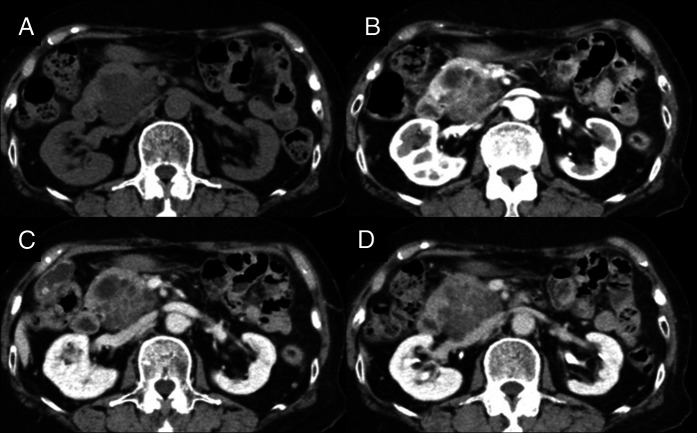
Abdominal dynamic computed tomography. (A) Plain scan, (B) late arterial phase, (C) portal venous phase, and (D) equilibrium phase. A 48 × 45-mm irregular multilocular cystic mass was observed in the pancreatic head. The central area appeared to contain clustered microcysts. The lesion showed maximal enhancement in the arterial phase, followed by washout.
